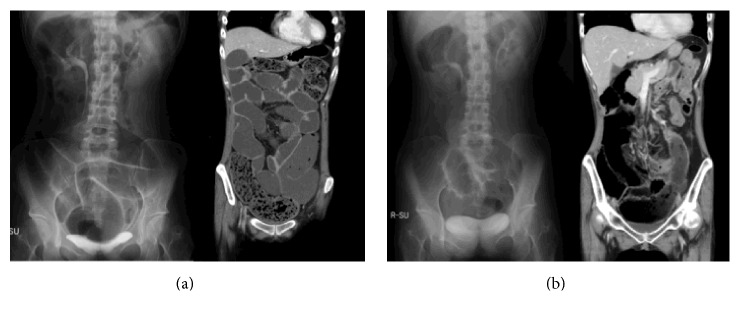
Abdominal radiography and CT findings at the emergent admission (a) and after treatment with octreotide (b). (a) Multiple and dilated small and large bowels with air-fluid levels in bowel loops (X-ray) and huge distention of bowel without mechanical obstruction (CT). (b) Thickening of the bowel wall and dilation of the bowels with air-fluid levels were improved.
